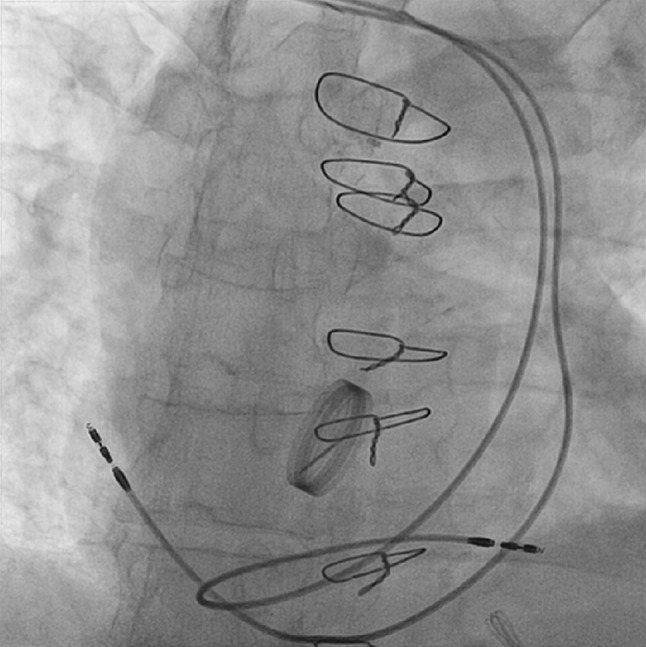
Radiograph showing final lead position in our patient
